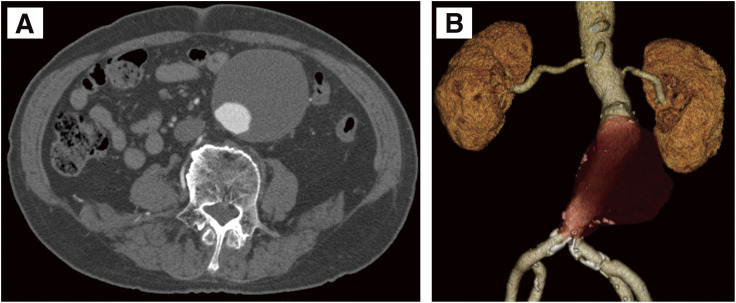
Preoperative contrast-enhanced computed tomography. (A) Axial image showing a maximum short-axis diameter of 65 mm. A large amount of mural thrombus is present within the aneurysm sac. (B) Three-dimensional reconstruction demonstrating a longitudinal length of 85 mm. The proximal neck measures 37 mm in length with an angulation of 55°, and the aneurysm sac contains extensive mural thrombus.
